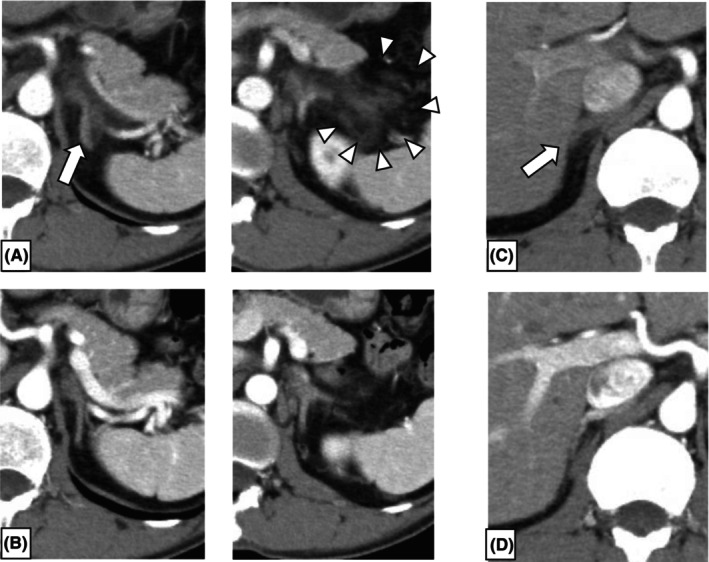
Contrast‐enhanced computed tomography (CT) showing development (A) and improvement of (B) the left adrenal infarction. Contrast‐enhanced CT showing development (C) and improvement (D) of the right adrenal infarction. (A) The arrow indicates hypertrophy of the left adrenal gland and non‐contrast‐enhanced areas, and arrowheads denote increased lipid concentrations and fluid retention around the left adrenal gland. The patient was diagnosed with left adrenal infarction. (B) Improvement of the left adrenal infarction. (C) The arrow indicates mild hypertrophy of the right adrenal gland. The patient was diagnosed with right adrenal infarction. (D) Improvement of the right adrenal infarction.
